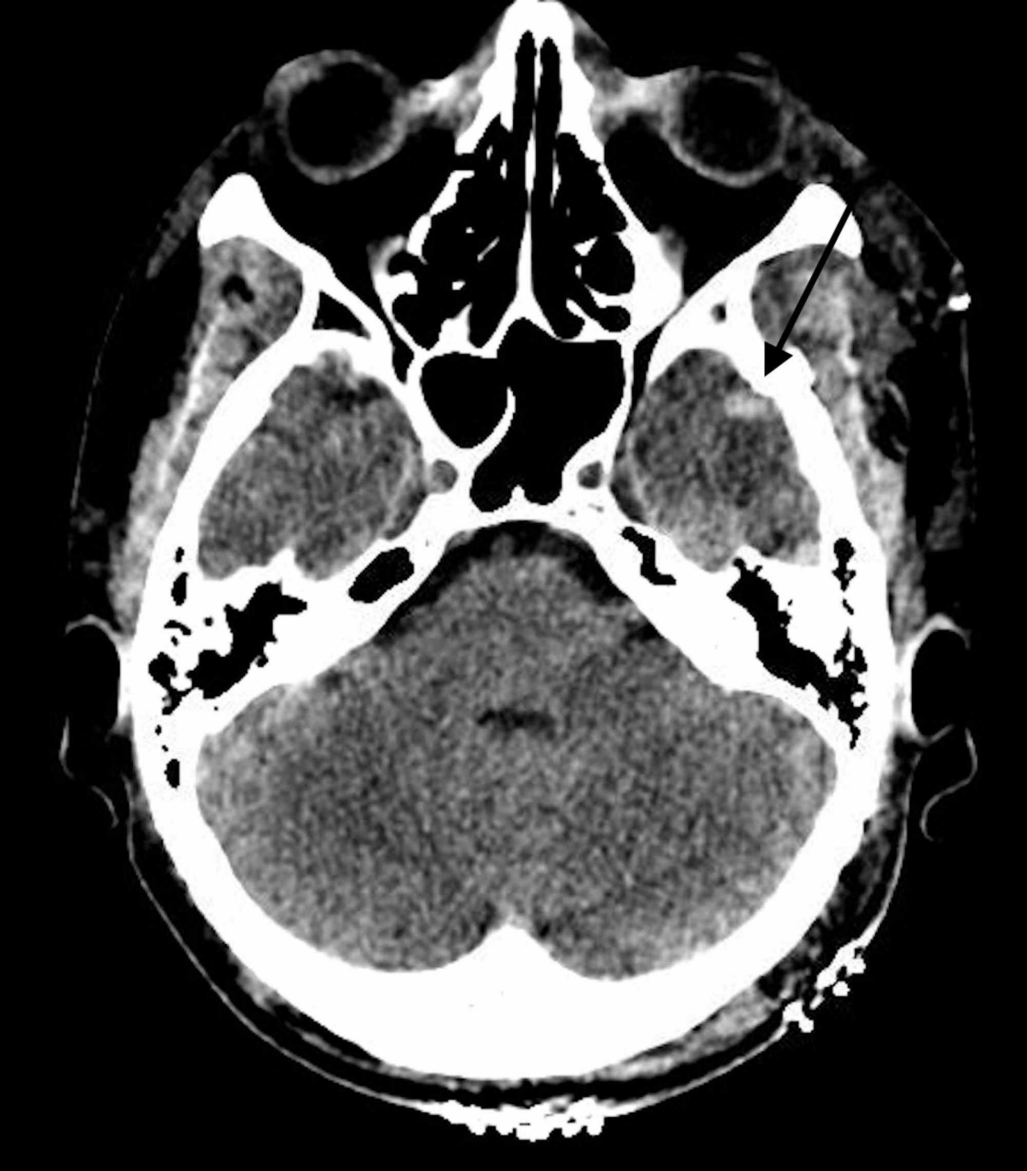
Postoperative CT of the head The image shows small left temporal lobe intracerebral hemorrhage (black arrow) CT: computed tomography
